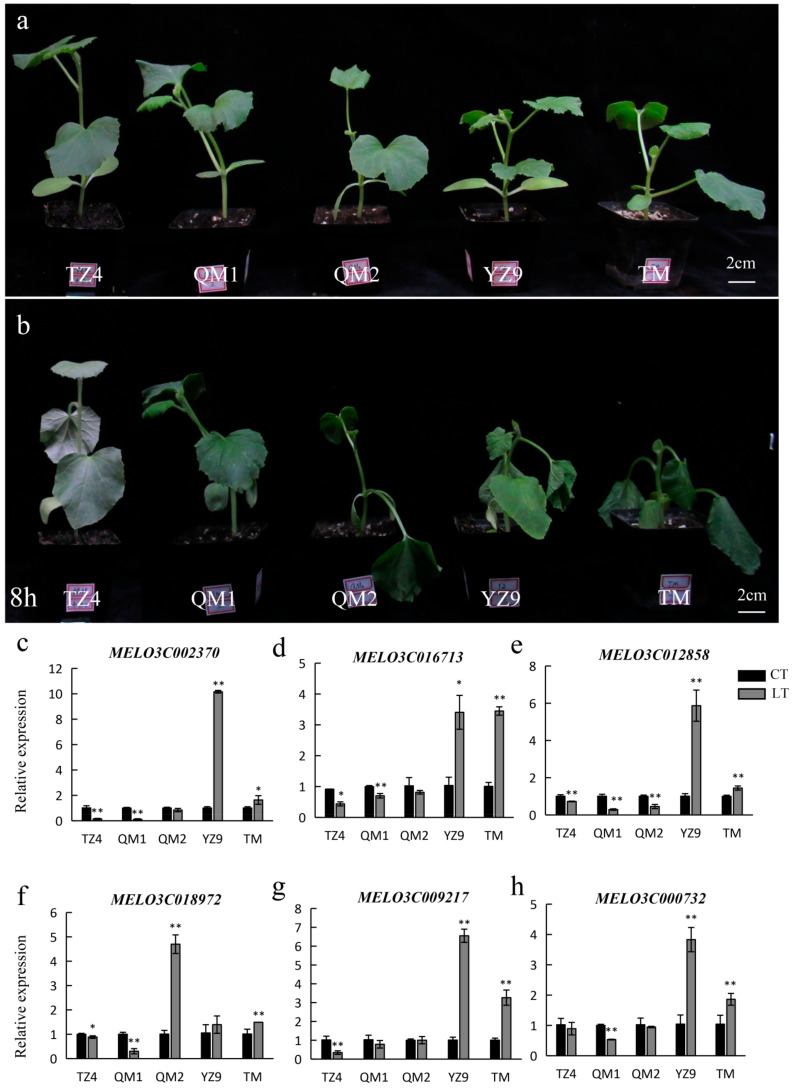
Expression analyses of key target genes in five cultivars of melon with different low-temperature resistance. (a,b) Phenotypic characteristics of five cultivars under low-temperature (4 °C) stress of 0 h (CK) (a) and 8 h (b). (c–h) Expression changes in six candidate target genes by low-temperature stress in cultivars of melon. CT and LT represent the normal (28 °C) and low-temperature (4 °C) treatments, respectively. Asterisks represent significant differences at the transcriptional level (Student’s *t*-test: *, *p* < 0.05; , *p* < 0.01).
